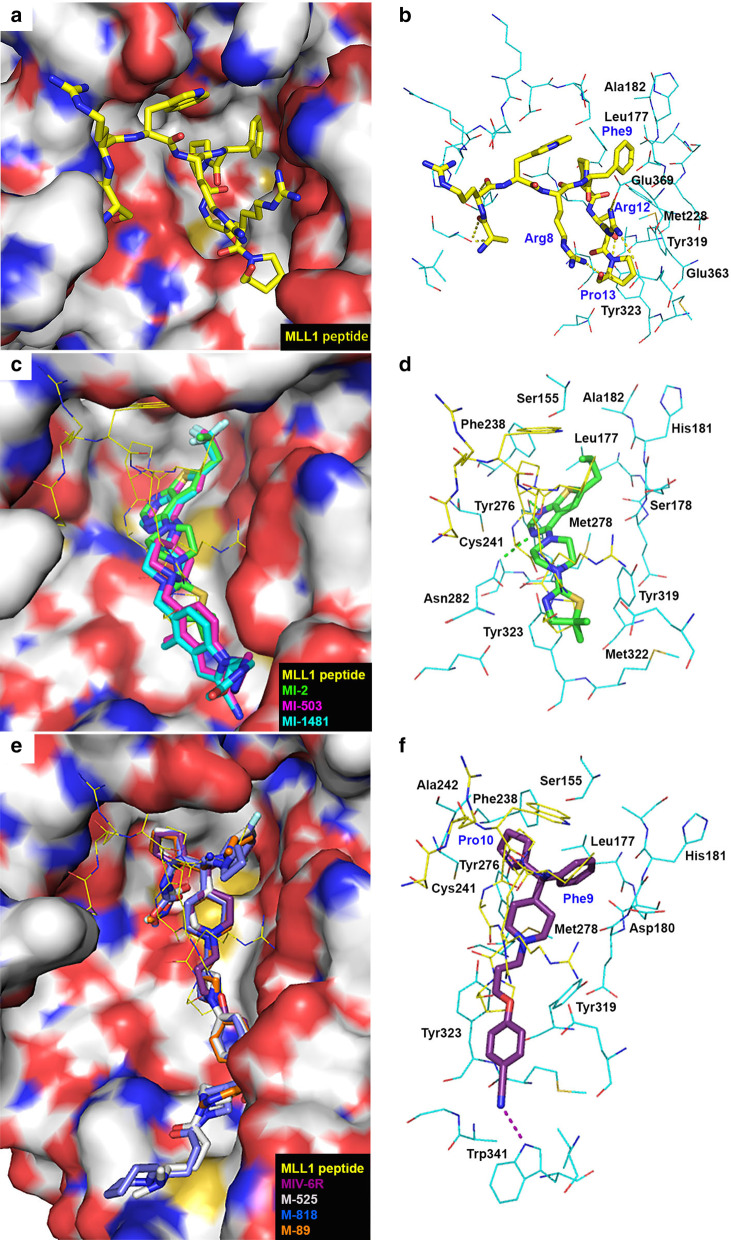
X-ray structures of Menin in complex with MLL1(6–13) peptide and inhibitors. a The active site of Menin–MLL1 complex (PDB: 3U85). b A close-up view of the Menin–MLL1 interaction. c Superimposed active sites of Menin–MLL1, Menin–MI-2 (PDB: 4GQ3), Menin–MI-503 (PDB: 4X5Y), and Menin–MI-1481 (PDB: 6BXY). d A close-up view of the Menin–MI-2 interactions. e Superimposed active sites of Menin–MLL1, Menin–MIV-6R (PDB: 4OG8), Menin–M-525 (PDB: 6B41), Menin–M-808 (PDB: 6WNH), Menin–M-89 (PDB: 6E1A). f A close-up view of the Menin-MIV-6R interactions. MLL1 peptide with C atoms in yellow is shown as a tube model in (a) and (b), while a line model in (c)–(f). Compounds MI-2, MI-503, MI-1481, MIV-6R, M-525, M-808, and M-89 are shown as tube models with C atoms in green, magentas, cyan, purple, grey, blue, and orange, respectively. Menin is shown as an electrostatic surface and hydrogen bonds are shown as dashed lines
